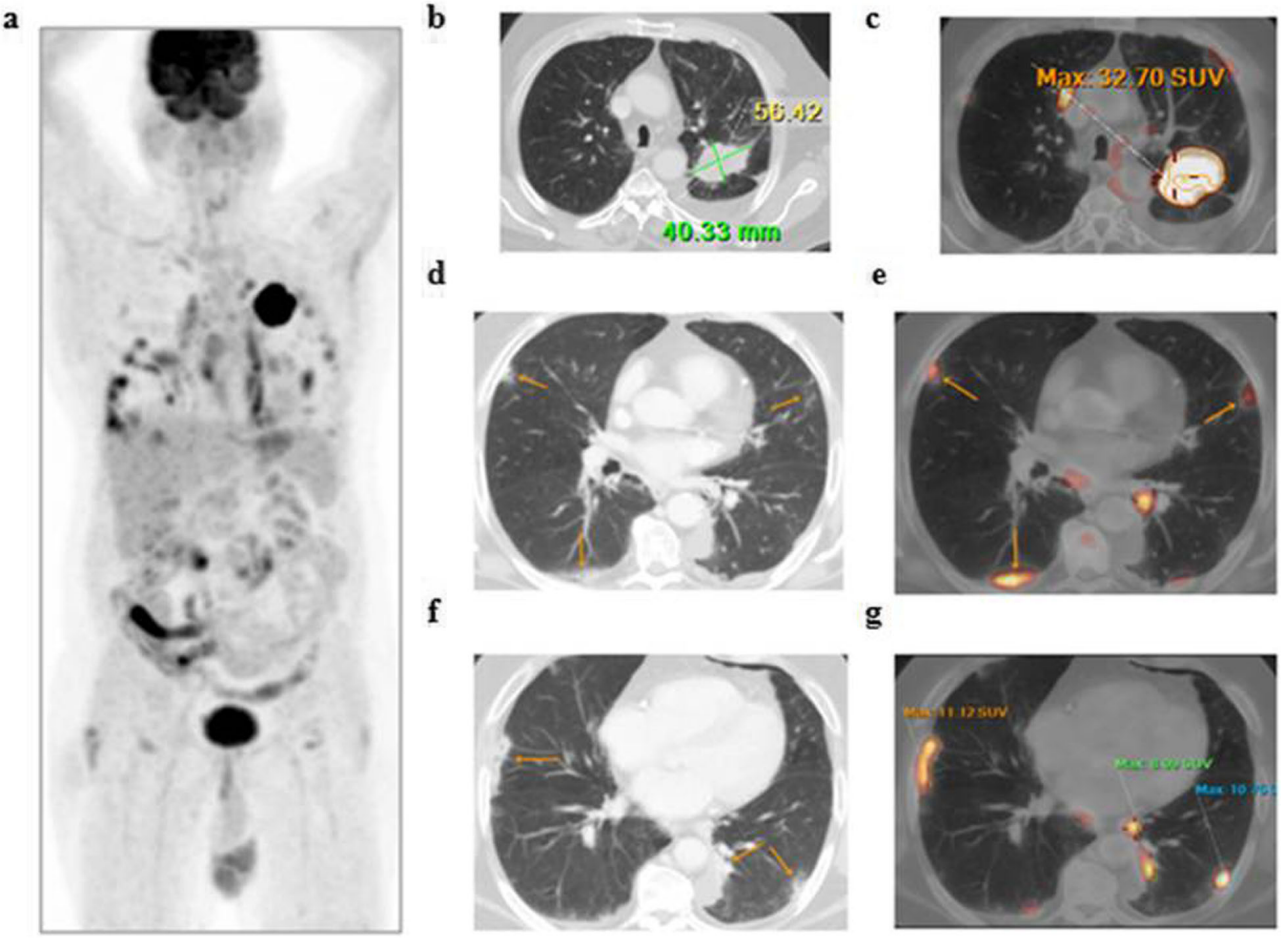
A 55-year-old male patient, presented with recently diagnosed left lung cancer and referred for initial PET/CT evaluation. a The whole-body MIP image shows an FDG avid upper left lung lesion with other multiple bilateral pulmonary metabolically active areas. Axial lung window CT (b) and corresponding fused PET/CT (c) images revealed an FDG avid left lung upper lobar speculated primary neoplastic mass (achieving 32.7 SUVmax), while axial CT images (d, f) revealed multiple bilateral subpleural variable-sized ground-glass opacities and patchy consolidation that show variable FDG avidity in corresponding fused PET/CT images (e, g) achieving up to 11.5 SUVmax
